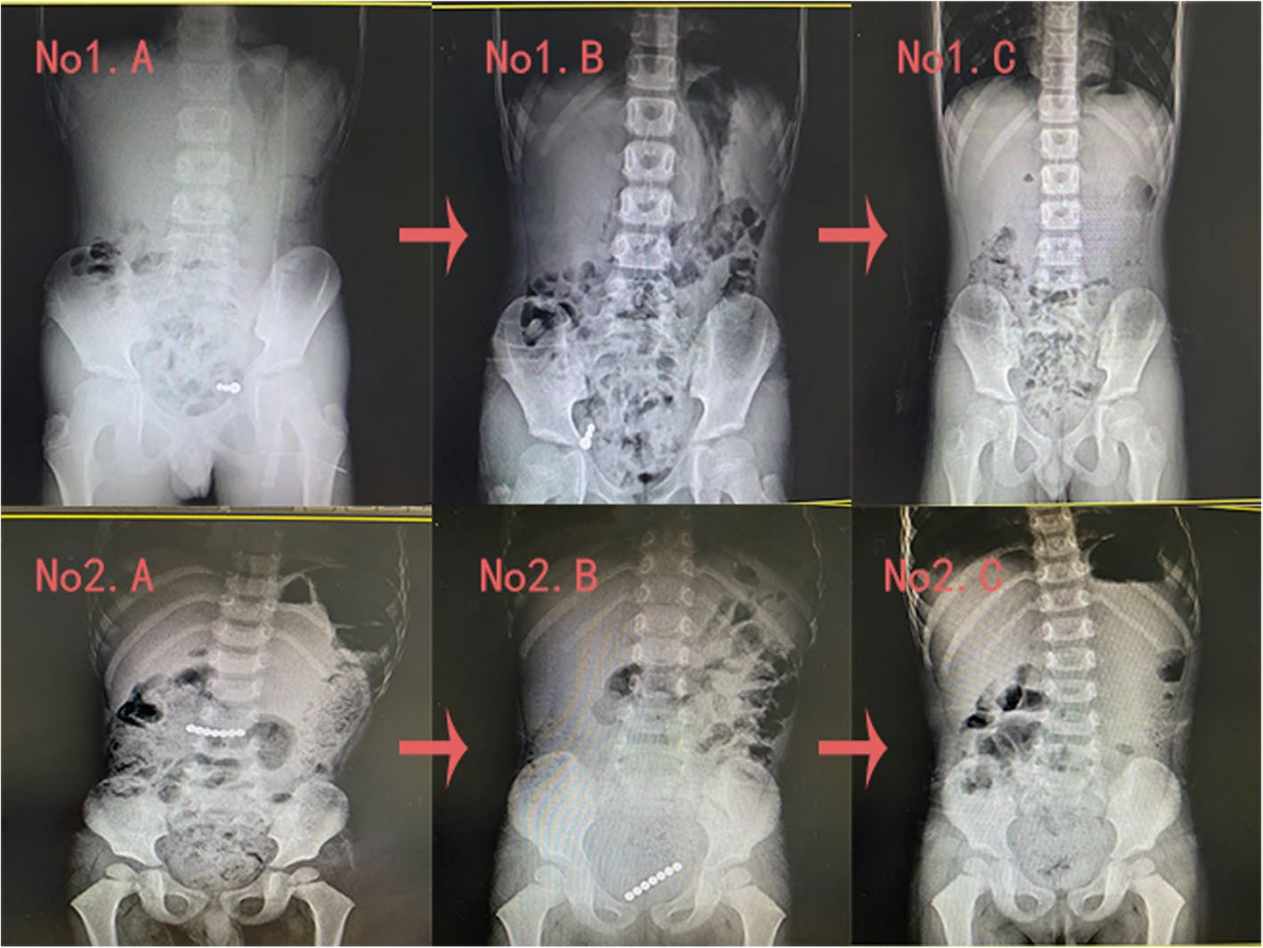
Patient 1 was asymptomatic and informed the doctor that the foreign bodies were swallowed at the same time. Serial x-rays showed that the foreign bodies moved and were excreted from the body. Patient 2 was asymptomatic and the doctor did not know if the foreign bodies were ingested at the same time. Serial x-rays showed a notable change in the position of the foreign bodies and they were discharged through the anus without medical interventions
